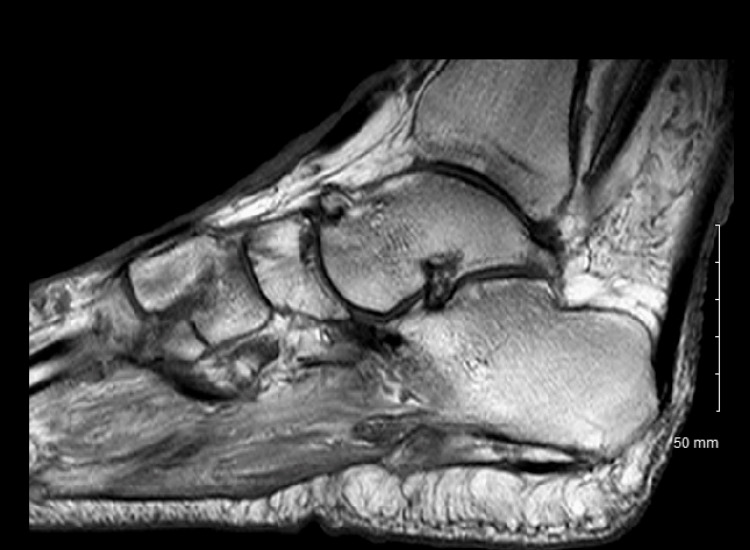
Lateral view MRI of the left foot.
